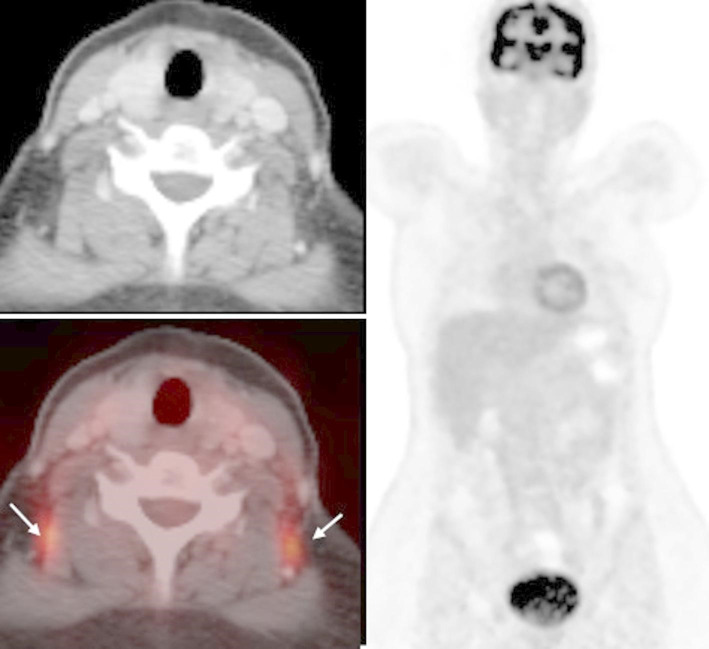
Follow-up PET-CT of the same patient with PTL Axial post-contrast CT (A), axial fused PET-CT (B) and coronal reformatted MIP image (C): complete metabolic remission mad morphologic resolution of all thyroid lesions and left cervical LNs with restoration of normal CT appearance and size of left thyroid lobe. Symmetrical intense FDG uptake in the paravertebral regions (short arrows), corresponding to fat areas without CT abnormalities compatible with activated brown adipose fat
